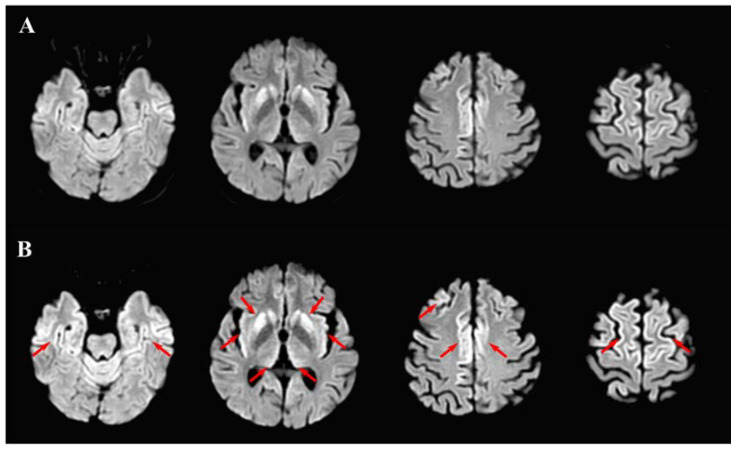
Brain MRI. Diffusion-weighted images displaying a hyperintense signal in the cortical mantle over the frontal, parietal, insular, and cingulate cortices, as well as bilateral putamina, caudate nuclei, and thalamus pulvinar before the SARS-CoV-2 infection (A). Repeated MRI performed nearly one month after the onset of SARS-CoV-2 infection was marked by a more enhanced signal (arrows) over the same regions (B).
